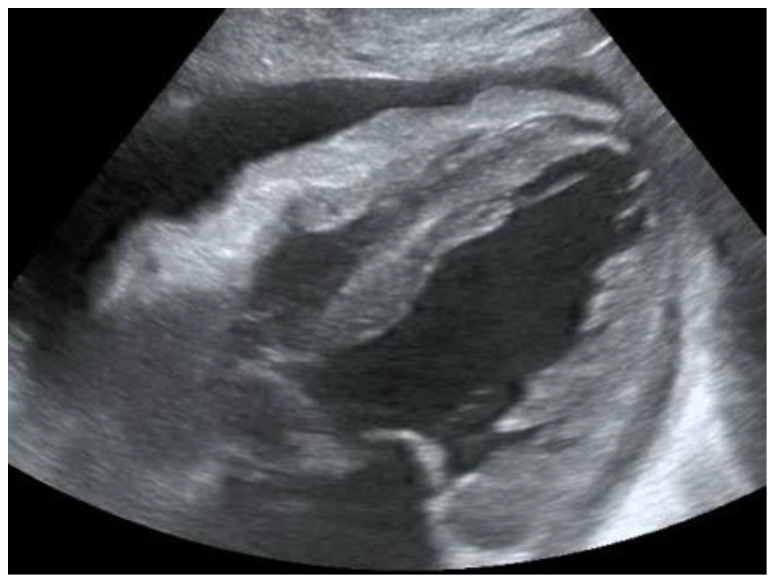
POCUS echocardiography: subcostal projection with pericardial effusion.
